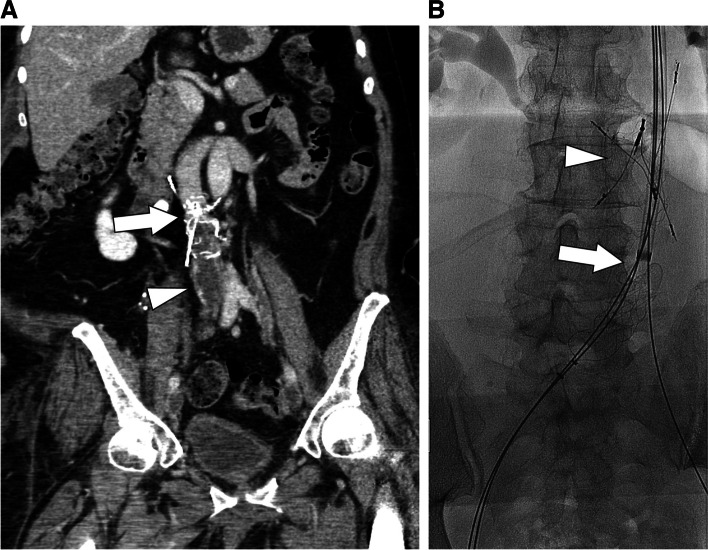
a. Coronal CT image demonstrating the Bird’s Nest filter (arrow) with thrombus extending peripherally below it (arrowhead). b. Frontal fluoroscopic image showing the ClotTriever deployed inside the right internal jugular sheath (arrow), avoiding the filter (arrowhead)
